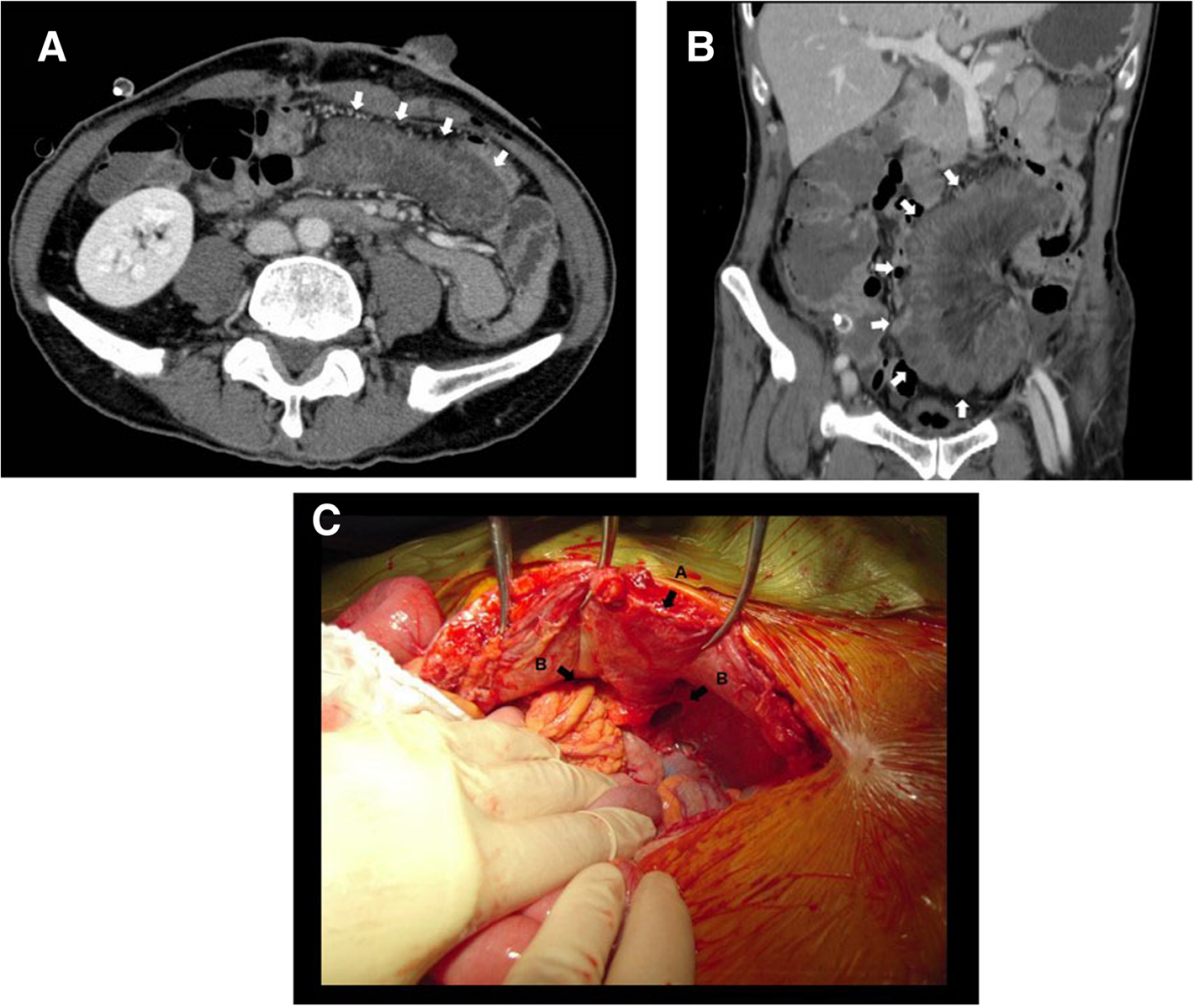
Emergency computed tomography and laparotomy findings after reduction of the herniated intestine. (A) and (B) After the onset of acute abdominal pain, the emergency computed tomography scan shows a loop of small intestine obstructed by the abdominal wall and sigmoid colon that resulted in sigmoidostomy (white arrows). The wall of the obstructed intestine is edematous and less enhanced. (C) The left side of the photograph is indicative of the cranial direction, and the right side shows the caudal direction. Sigmoidostomy is indicated by the black arrow marked A, and the route of herniation through the space lateral to the sigmoid colon is indicated by the black arrows marked B.
